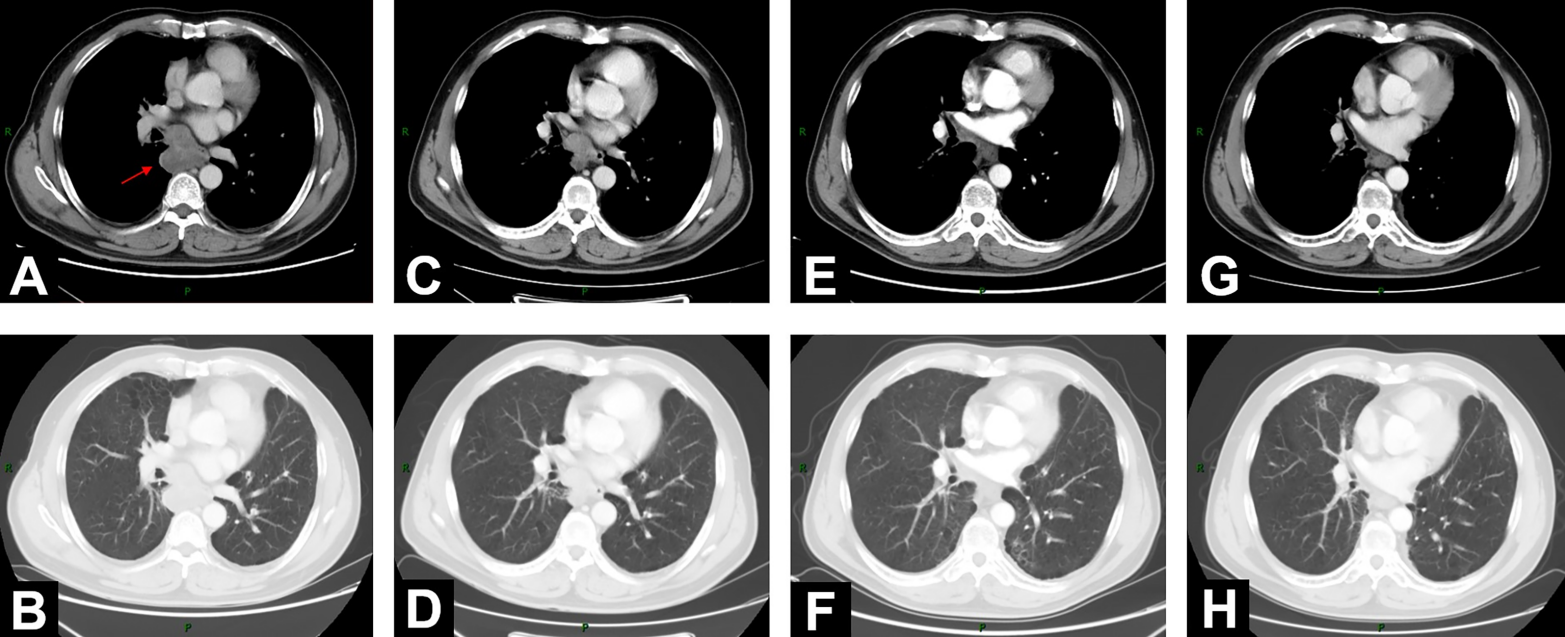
Changes of mediastinal lesion on chest contrast-enhanced CT scans. (A, B) On June 18, 2020, CT demonstrated enlarged mediastinal lymph node station 7 with heterogeneous enhancement (5.2 cm * 4.7 cm). (C, D) CT on August 11, 2020, after 2-cycle induction chemotherapy (3.9 cm * 2.9 cm). (E, F) CT on November 13, 2020 (2.0 cm * 1.5 cm), 1 month after concurrent chemoradiotherapy (cCRT). (G, H) At 1-year follow-up after consolidation durvalumab, CT on October 26, 2021 (1.6 cm * 1.3 cm).
